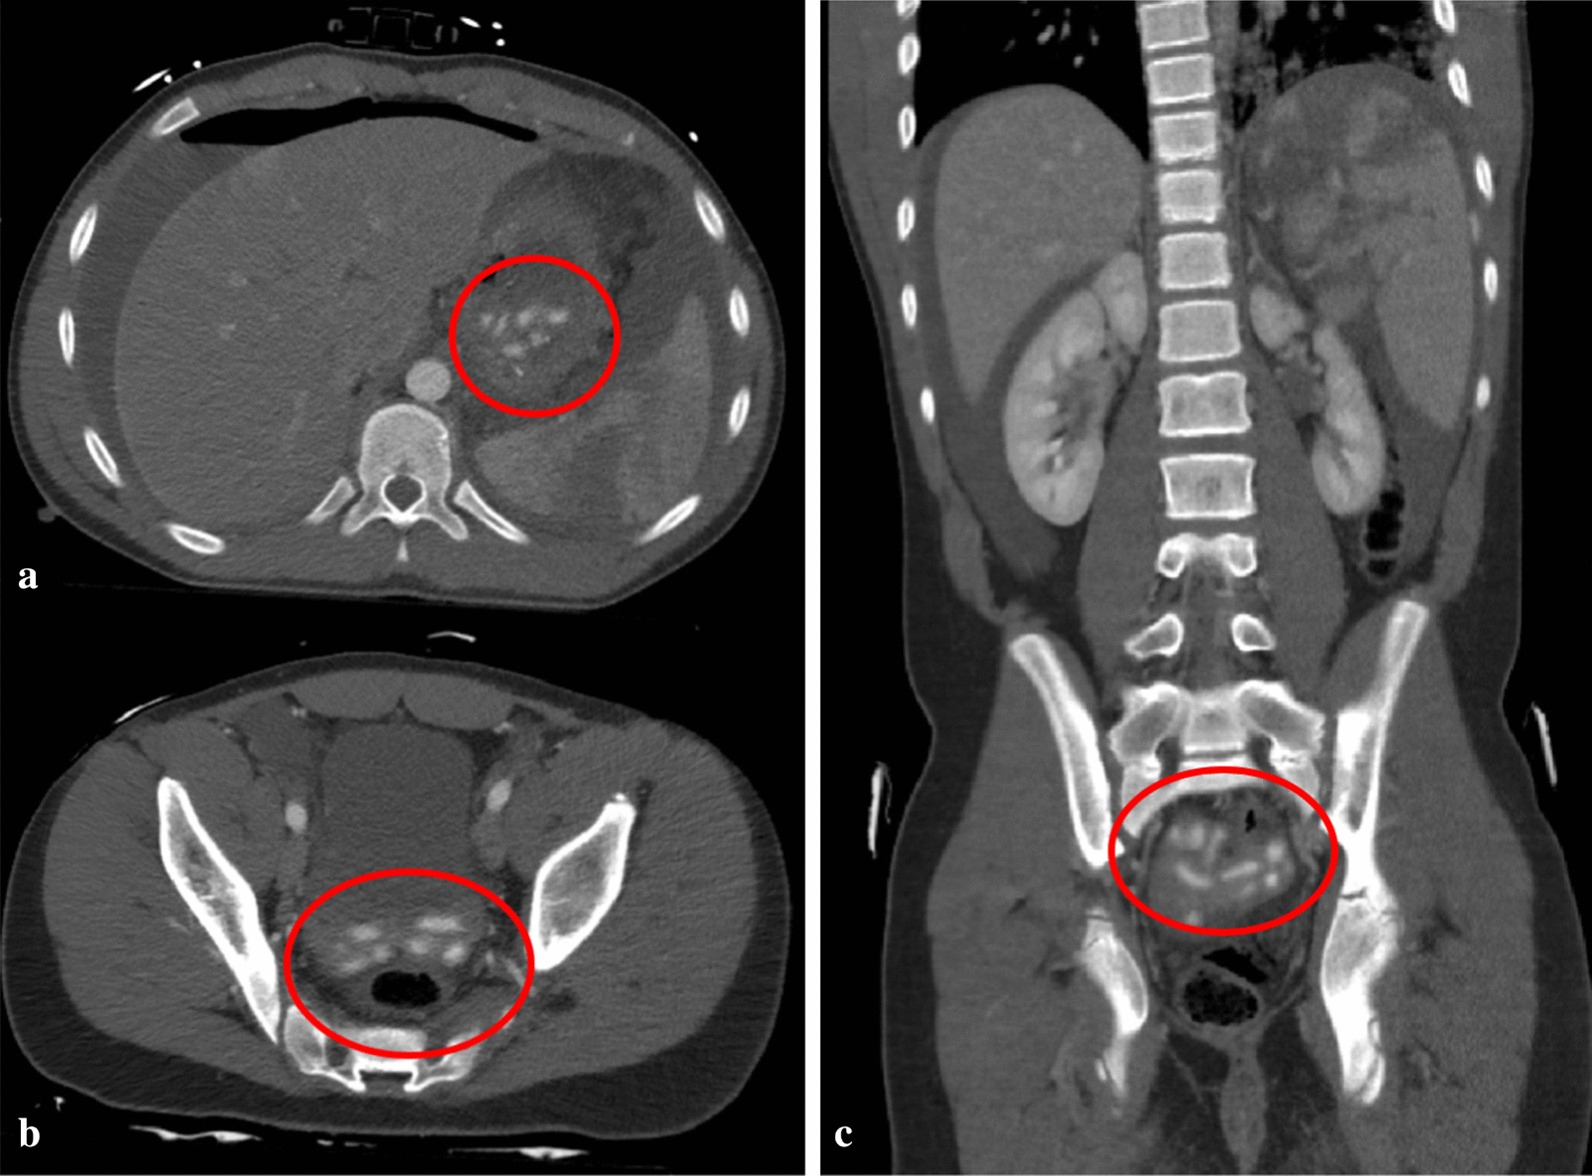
Computed tomography (CT) of the abdomen after blunt abdominal injury due to a motocross accident. a Axial contrast enhanced CT image of the abdomen at the portal venous phase shows free abdominal fluids, free air, a spleen laceration and remarkable spherical shaped corpora aliena in the stomach; b axial image, demonstrating the same hyperdense lesions in the pelvic cavity; c coronal image, showing a grade 4 splenic rupture as well as hyperdense lesions in the pelvic cavity
